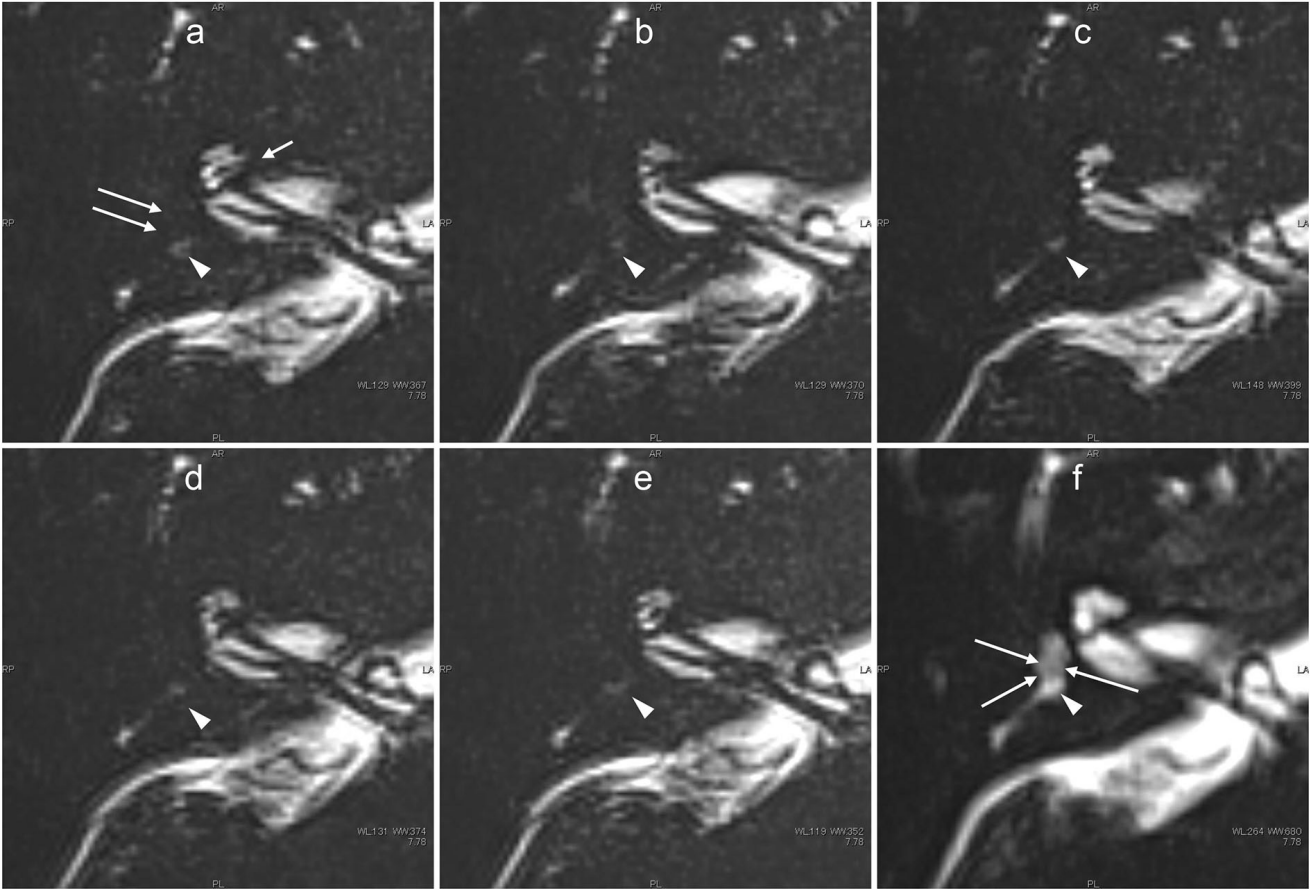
Images obtained from the male patient aged in his 40's with a history of acute low-tone sensorineural hearing loss 3 years previously but no history of vertigo who was currently asymptomatic. 3 years earlier, the patient had been shown to have cochlear endolymphatic hydrops by previous contrast-enhanced MR imaging using an intravenous gadolinium-based contrast agent. In this patient, five ultra-heavily T2-weighted images (6 min scan with a TE of 3,200 ms) were obtained serially (a–e), and a 3 min scan image with a TE of 540 ms was obtained as a less sensitive scan to delineate the labyrinthine anatomy (f). From the initial scan obtained ~30 min after intratympanic administration of ^17^O-labeled water (a), the signals for the vestibular signal (arrows) and anterior part of the basal turn in the cochlea (short arrow) were almost completely lost because of the distribution of ^17^O-labeled water. Note that most of the vestibule showed a slightly decreased signal even in the less sensitive image with a TE of 540 ms (arrows, f). The concentration of intralabyrinthine ^17^O-labeled water was higher in this patient than in the volunteers. The signal for the posterior ampulla (arrowheads in a–f) decreased gradually from the initial phase (a) to the third phase (c). From the third phase (c) to the fifth phase (e), almost no signal was observed in the posterior ampulla.
